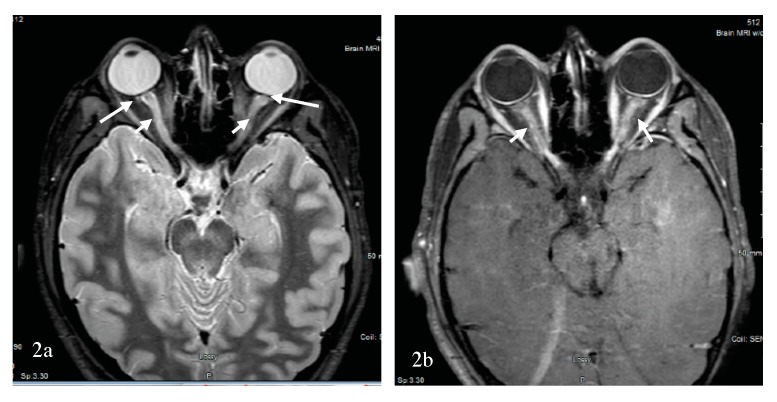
Axial short tau inversion recovery (STIR and T1 with contrast orbital MRI of an 18-year-old Caucasian male, with bilateral longitudinally extensive (small arrows) optic neuritis with anterior predominance, perineural sheath swelling (long arrows) and tilting and twisting of both optic nerves better seen on axial STIR (2a,2b). Serum MOG-antibody was positive.
